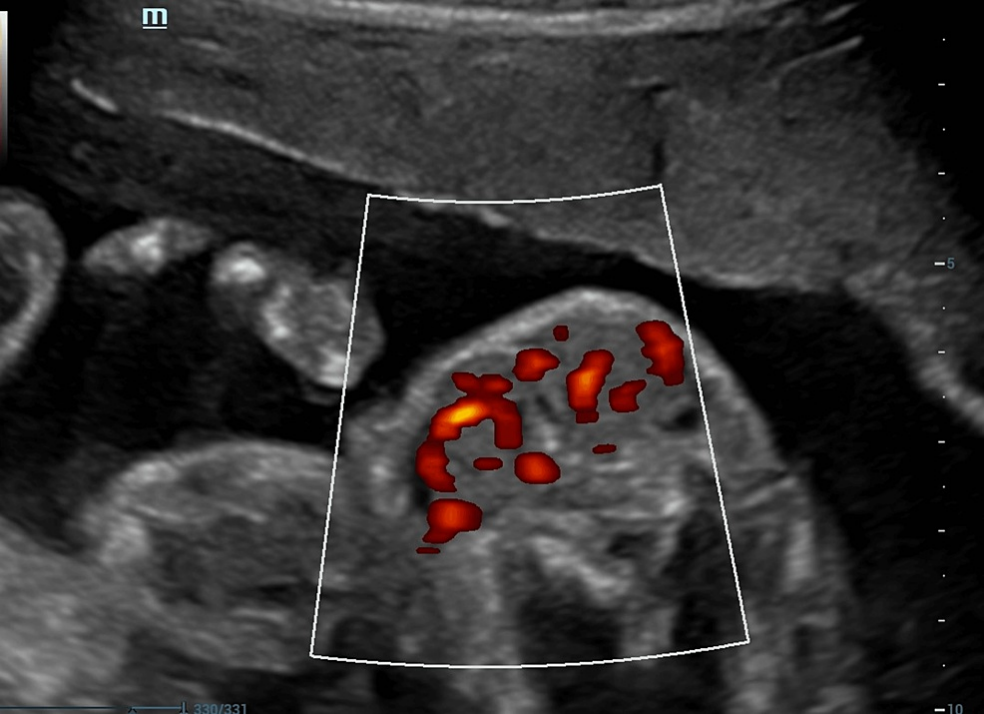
Color Doppler of the fetal goiter
